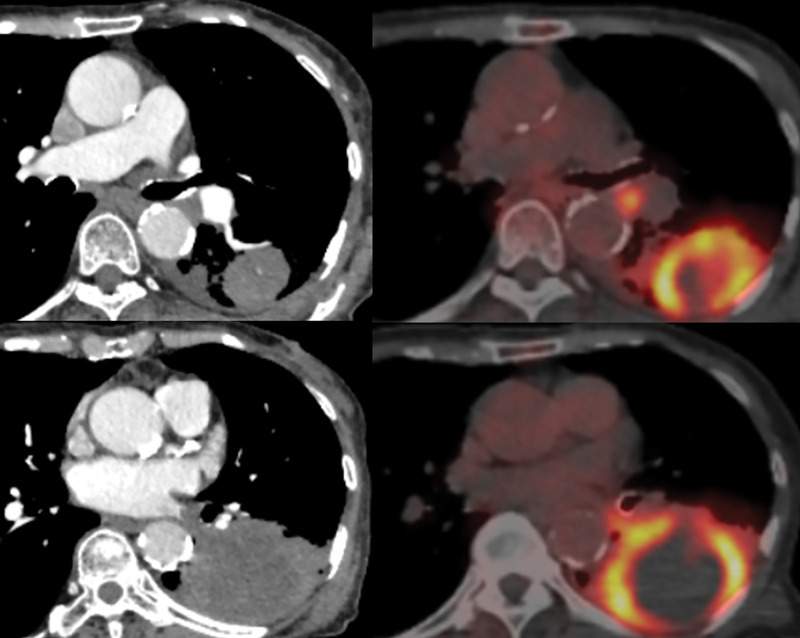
CT and PET images showing a large tumor in the left lower lobe of the lung and enlarged hilar lymph nodes with strong uptake of fluorodeoxyglucose. Tumor invasion of the left atrium is evident.
